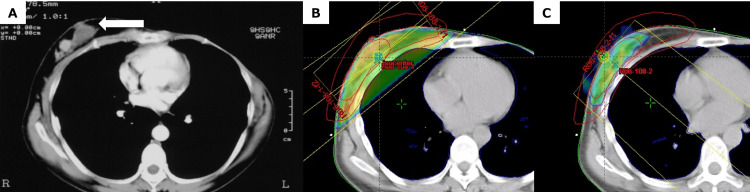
CT image at the time of diagnosis of right breast cancer, and radiation therapy field after the surgery for right breast cancer In Figure A, the arrow (←) shows the right breast cancer, after mastectomy was performed on left breast cancer. Figure B and C shows the radiation field after surgery for right breast cancer. In Figure B, the colored area shows the tangential irradiation field. In Figure C, the colored area shows the boost irradiation field.
